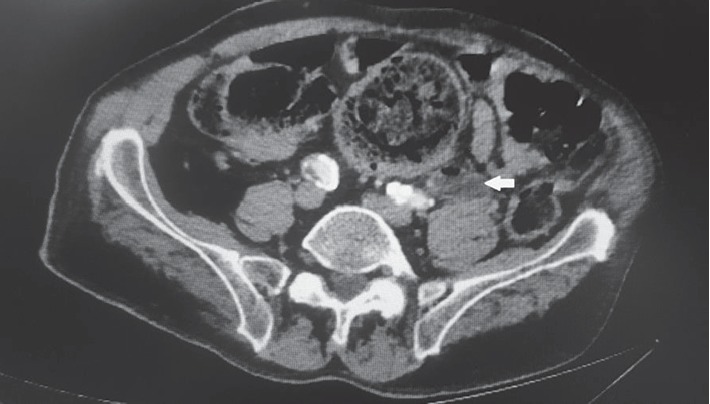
Computerized tomographic (CT) scan of the abdomen and pelvis. Left hydroureter with presence of intraluminal air (arrow).
